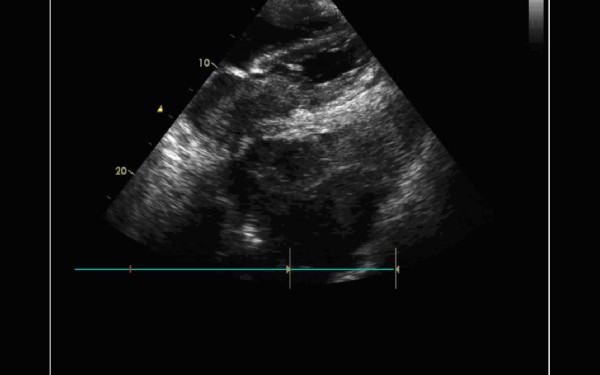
Sonography with a large mass in the left pleural space.
